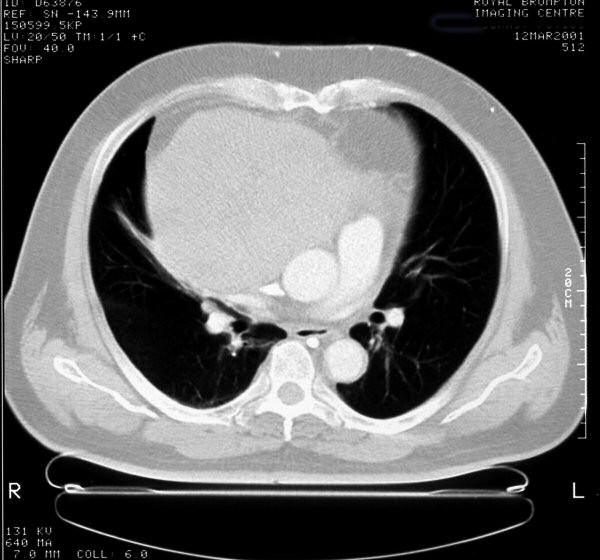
A pre-surgical CT scan of the chest showing the tumour.
